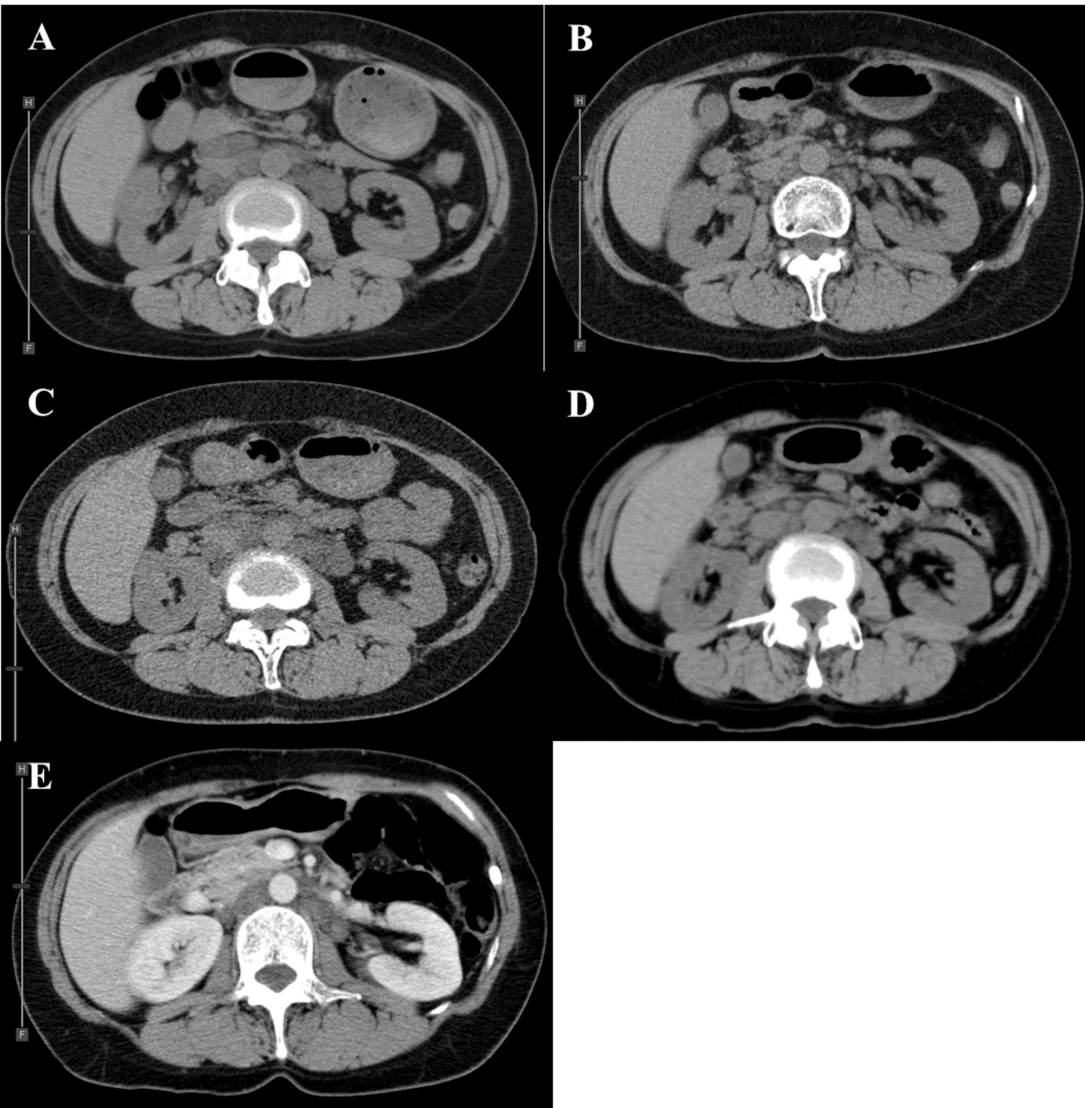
Changes in CT findings of the abdominal lesion over time prior to presentation at our hospital. (A) Initial findings when the abdominal lesion was first identified at another hospital (seven years before presentation); (B) Findings six years before presentation at our hospital: the abdominal lesion had decreased in size; (C) Findings five years before presentation at our hospital: the abdominal lesion had slightly increased in size; (D) Findings four years before presentation at our hospital: the abdominal lesion had decreased in size again; (E) Findings three years before presentation at our hospital: the abdominal lesion had increased in size again.
